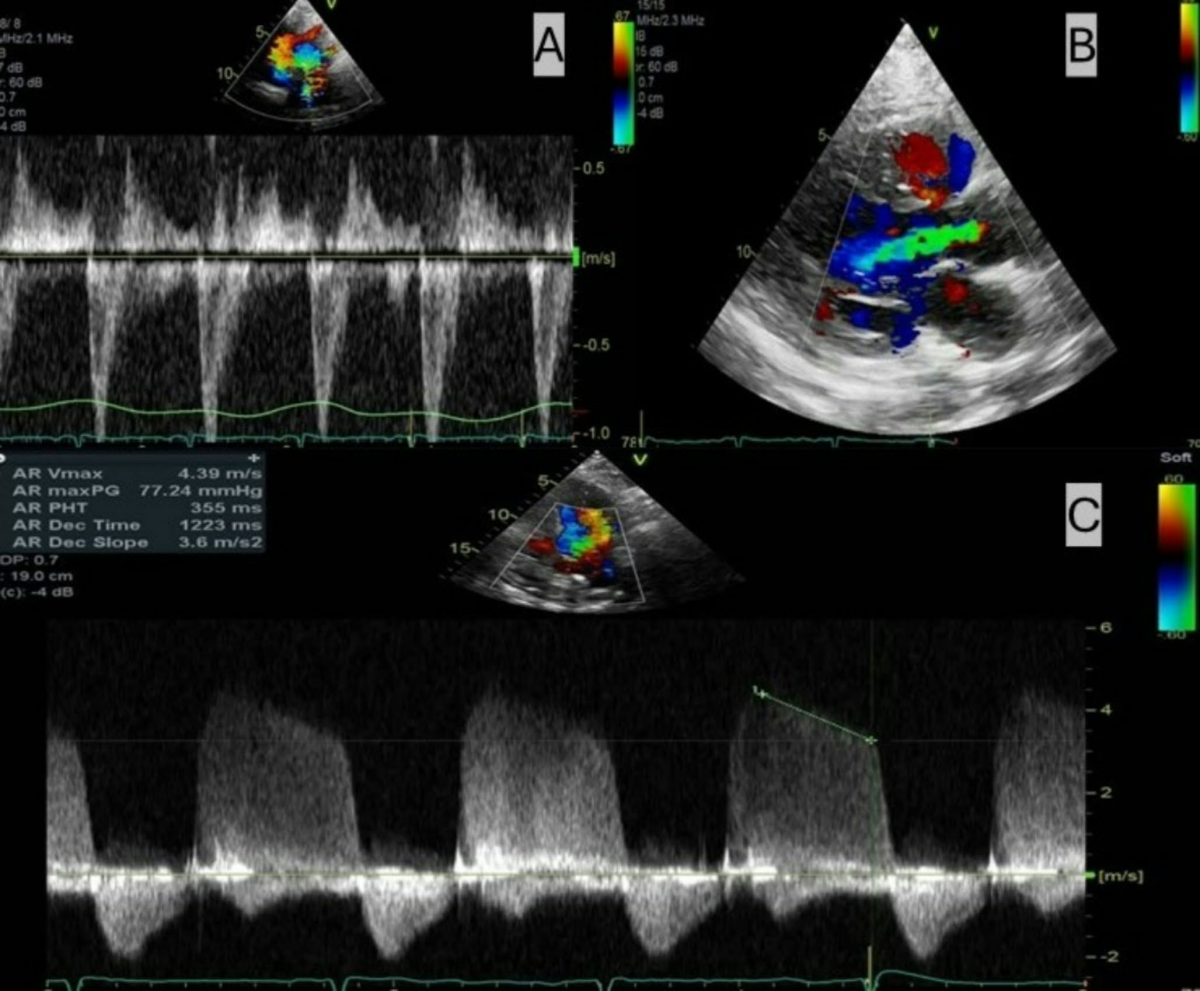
Transthoracic Doppler analysis. (A) suprasternal view showing holodiastolic flow reversal of the descending aorta; (B) parasternal long-axis showing vena contracta of 5.5 mm; (C) apical 5-chamber view showing aortic regurgitation with pressure half-time of 355 msec
